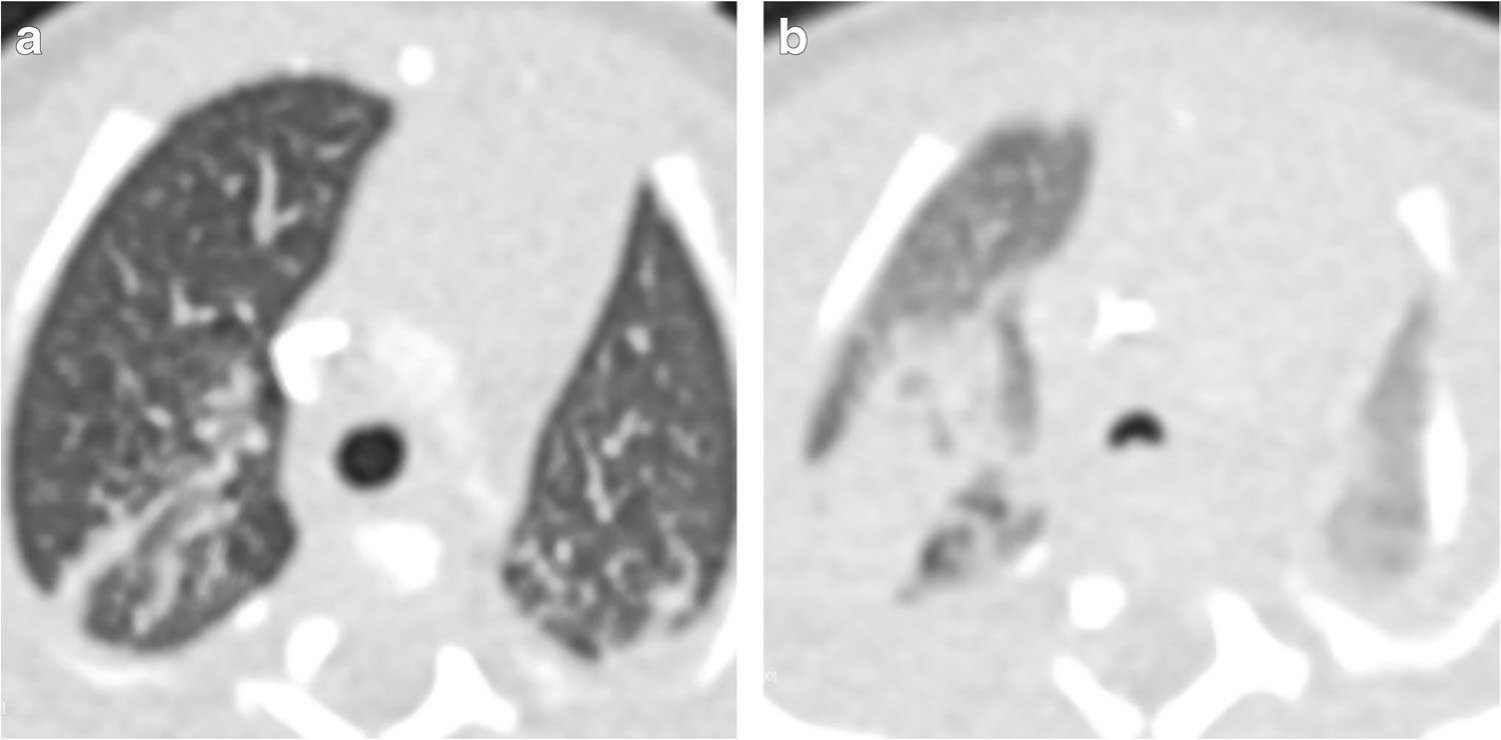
Airway pressure imaging in a preterm boy born at 25 weeks of gestation, now post-menstrual age 31 weeks (6 weeks old). a, b Axial CT demonstrates the difference in the appearance of the lungs and trachea when imaged with 14 cm of water-positive end-expiratory pressure (PEEP) (a) and without PEEP (b). Airway pressure support is often needed but can mask tracheobronchomalacia when it is present. Removing the pressure support at the time of imaging can be very helpful in evaluating the lungs and the airway when performing CT
